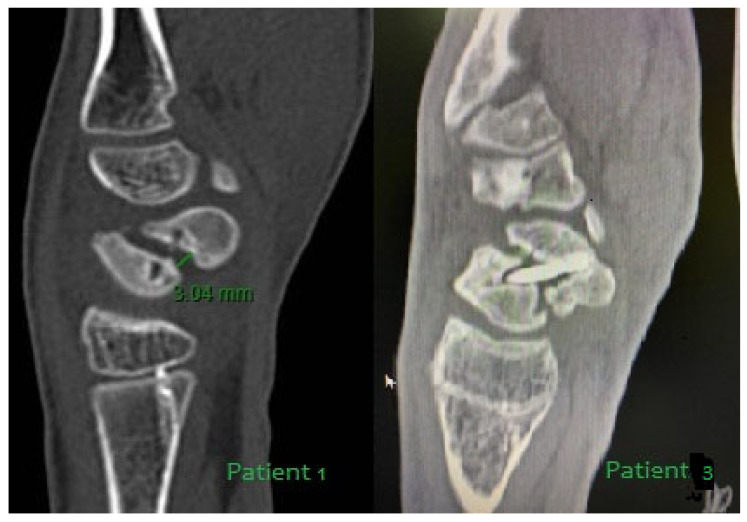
CT scans of Patients 1 and 3 revealed a fracture gap with significant bone resorption. In Patient 3, the non-union had already been fixed with a screw.
